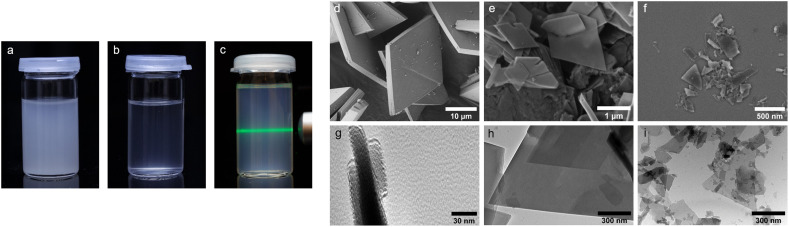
Dispersions of Zr-bzpdc-MOF micro flakes in acetone: (a) micro flakes 1 direct after preparation and (b) after 24 h; (c) downsized flakes 2 after 6 months. SEM images of Zr-bzpdc-MOF as (d) single crystals, (e) micro flakes 1 and (f) micro flakes after ultrasonication 2. TEM images of micro flakes 1 in (g) cross section and (h) top view; (i) TEM image of micro flakes after ultrasonication 2.
